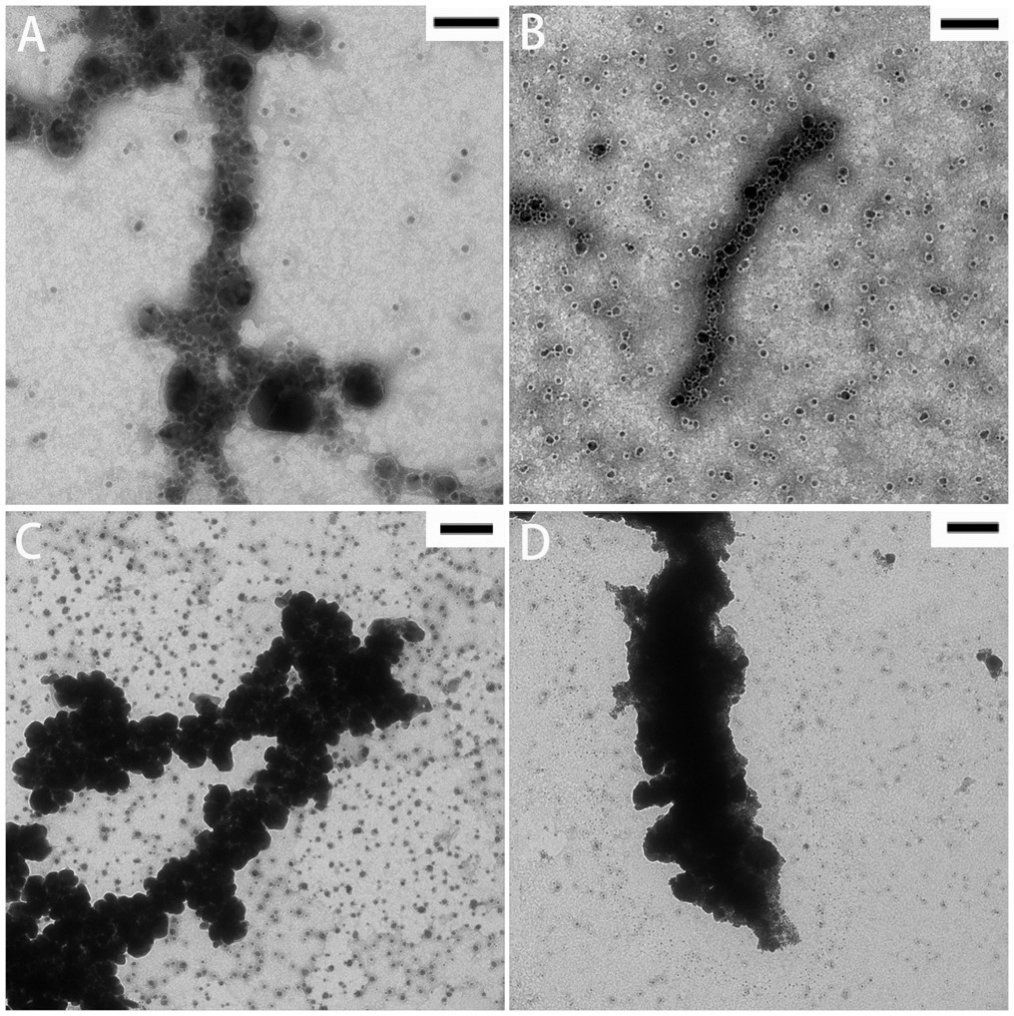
TEM images of silver-enhanced SBAFP-CT fibrils. A & B) Shorter gold-labelled fibrils with a small amount of silver enhancement. C & D) Longer gold-labelled fibrils with strong silver enhancement. Scale bar: A) 60 nm; B) 100 nm; C & D) 200 nm.
